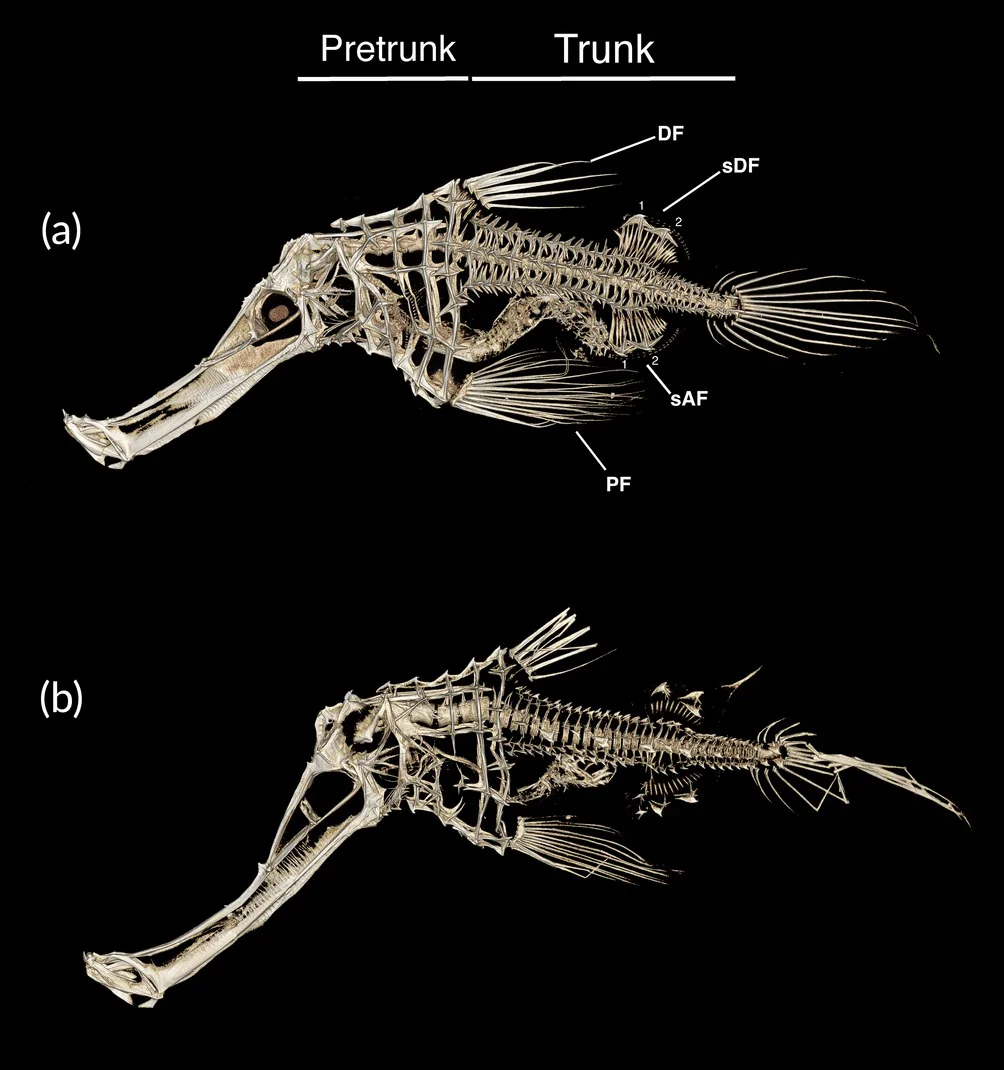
Micro‐CT reconstructions (lateral view) of *S. snuffleupagus*: (a) holotype, female, AMS I.33751‐047; (b) paratype, male, NTM S.13600–047. DF, dorsal‐fin; PF, pectoral‐fin; sAF, soft anal‐fin; sDF, soft dorsal‐fin. Numbers 1 and 2 indicate the respective pterygiophores supporting the soft dorsal‐ and anal‐fins.
